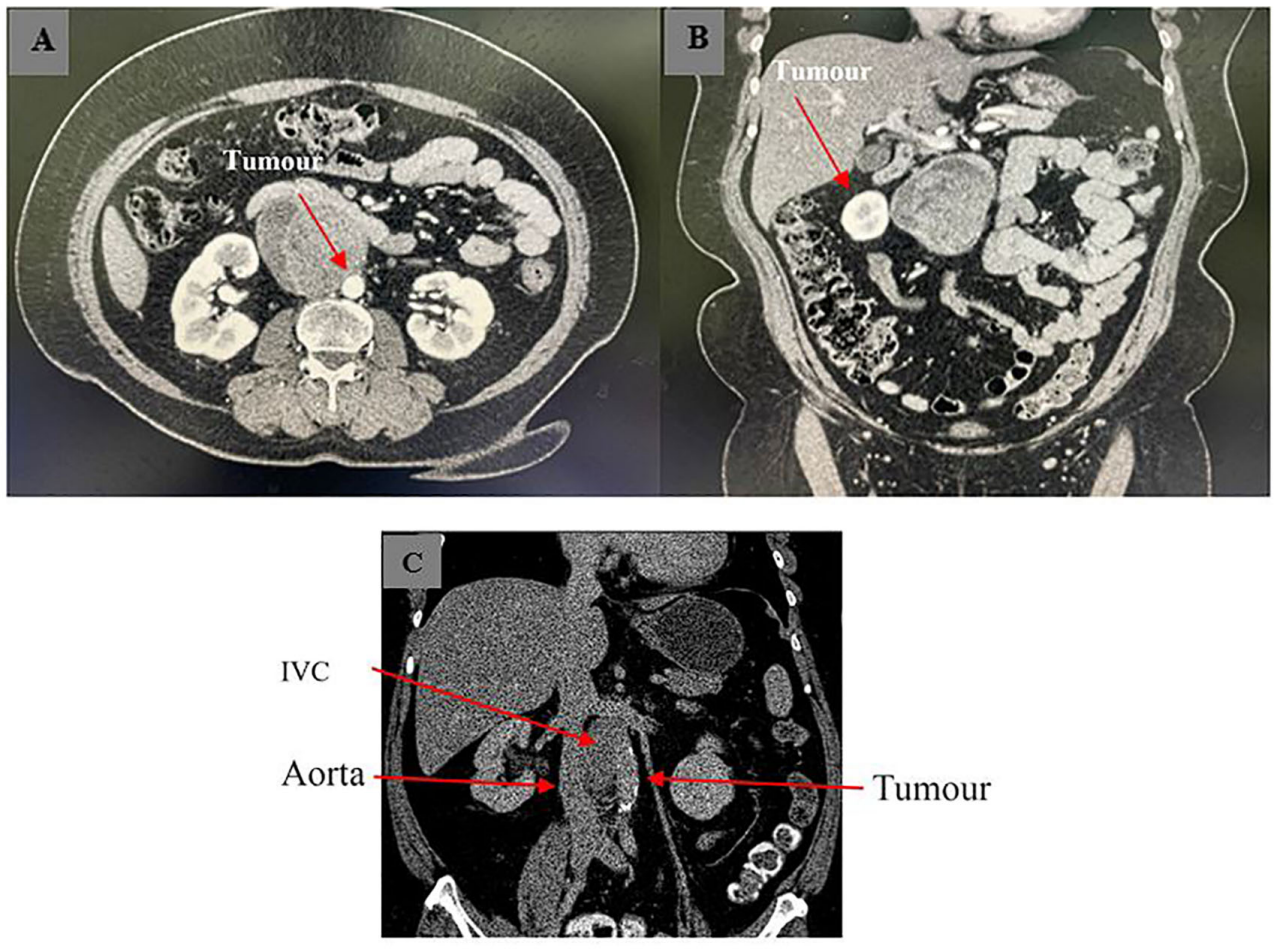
Axial (A, B) and coronal view (C) of CT imaging showing retroperitoneal tumor with close proximity to major vessels. The tumor appeared as a hypodense mass under CT imaging, suggesting necrosis within the mass.
